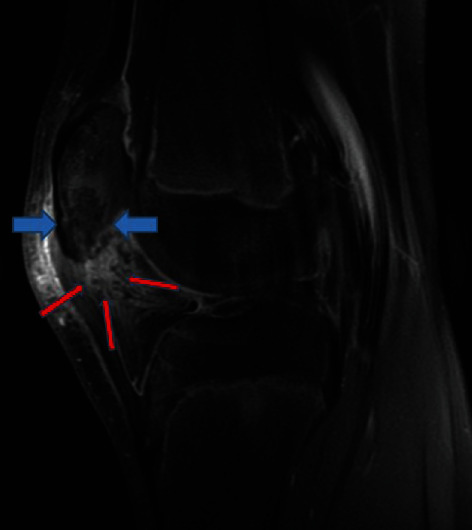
MRI 3. Sagittal T1 fat sat view. Osteomyelitis of the patella (blue arrows) and fat pad infiltration were observed (red arrows).
